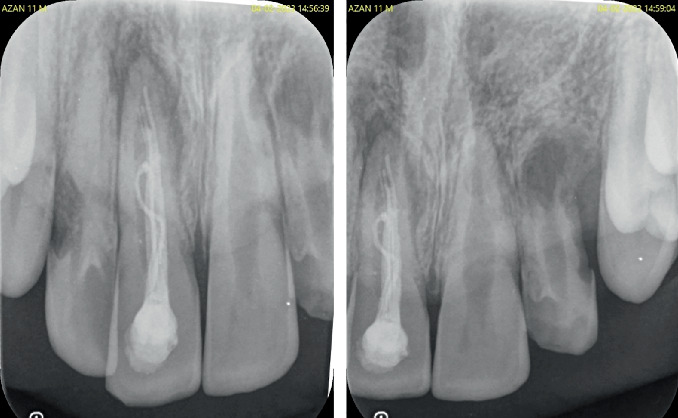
Pre-treatment intraoral periapical radiographs in relation to Tooth Numbers 12, 11, 21 and 22.
